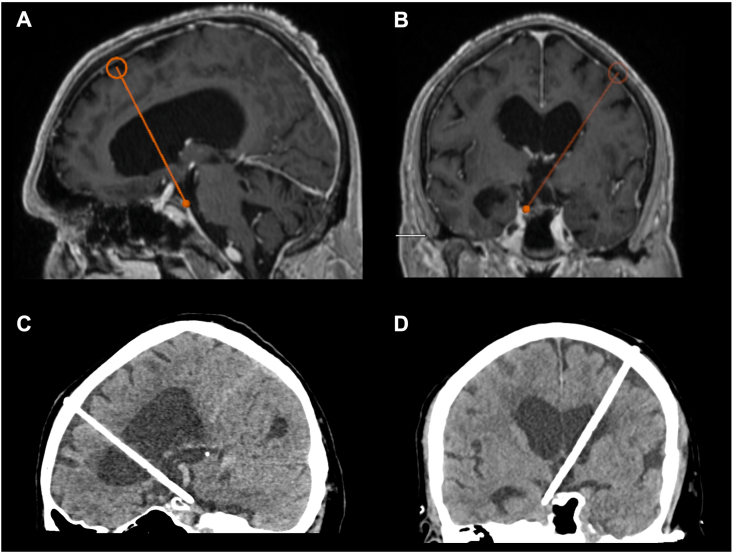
T1-weighted with contrast cMRI scans of the preoperative trajectory planning for an STS in a patient with aqueductal stenosis due to an arachnoid web vie a left frontal trajectory (sagittal and coronal, A and B). Postoperative cCT with the internal shunt catheter tip in the prepontine cistern (sagittal and coronal, C and D).
